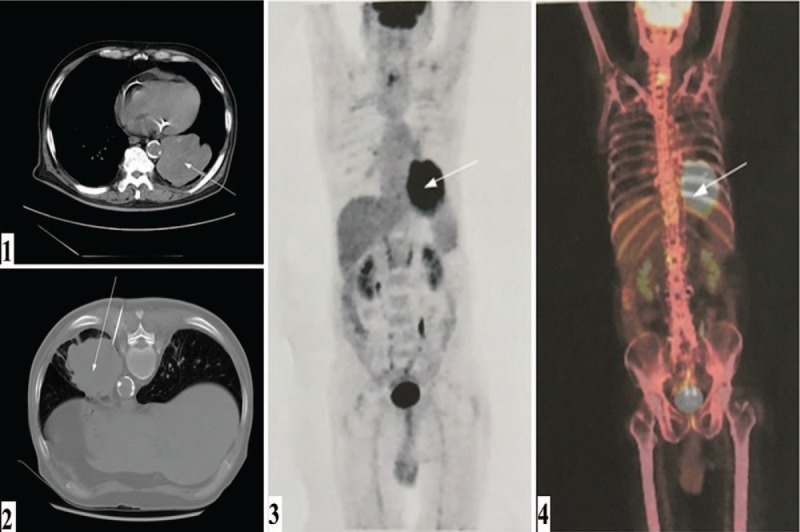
The patient's imaging and pathological findings. Thoracic enhanced computed tomography scan (1, 2). Positron emission tomography-computed tomography imaging (3, 4).
